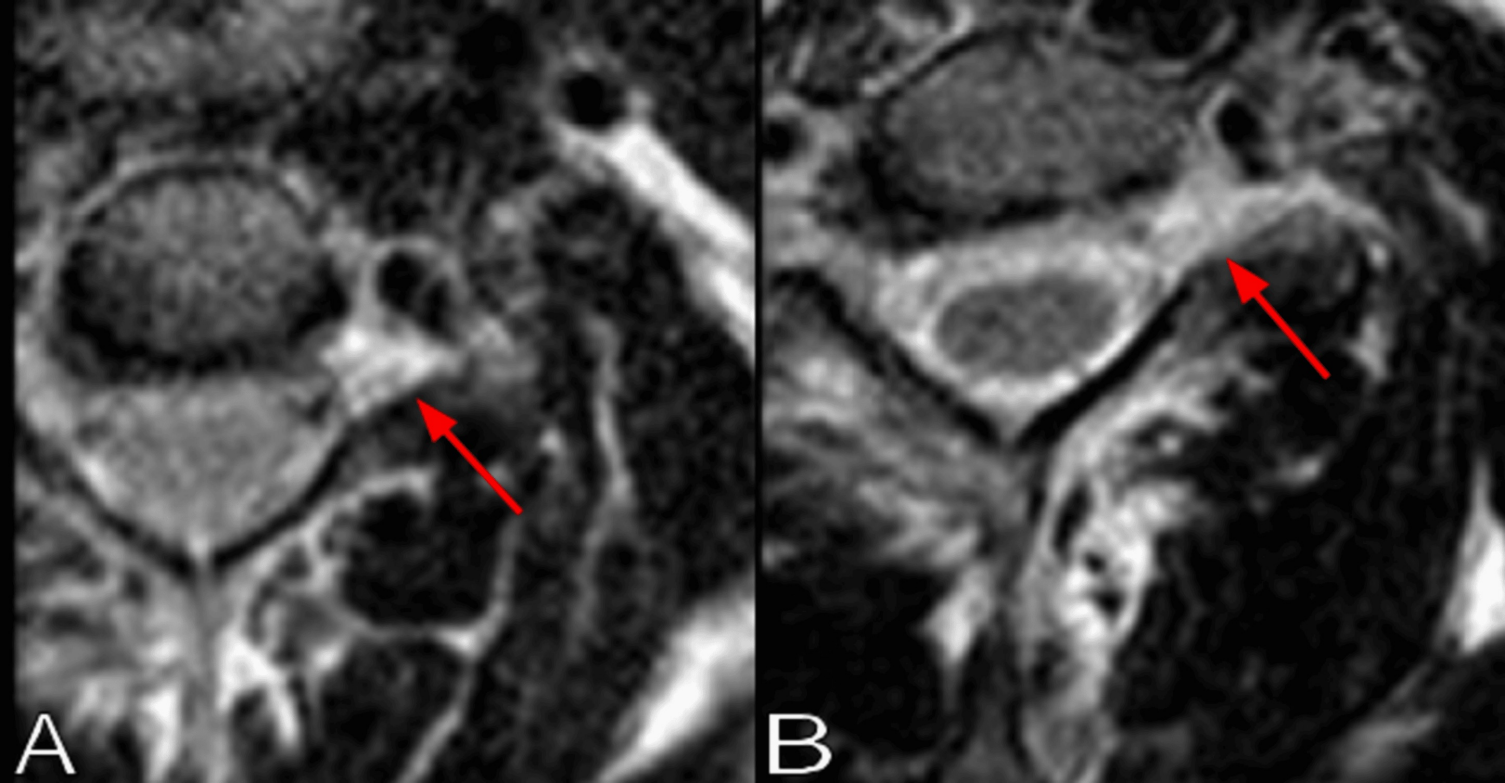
Brachial plexus axial MRI T2-weighted images showing (A) left C4-C5 and (B) left C5-C6 nerve root sleeve tears (red arrows).
